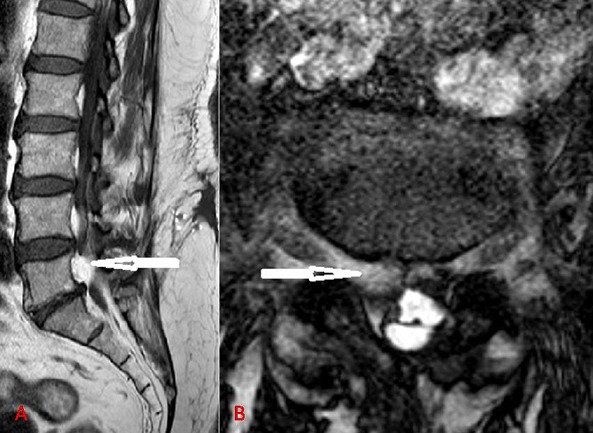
A) T2-weighted magnetic resonance (MR) sagittal images of the right paracentral area showing a mass (arrow) in the spinal canal at L5; B) T2-weighted axial magnetic resonance images at the L5-S1 neural foramen level
